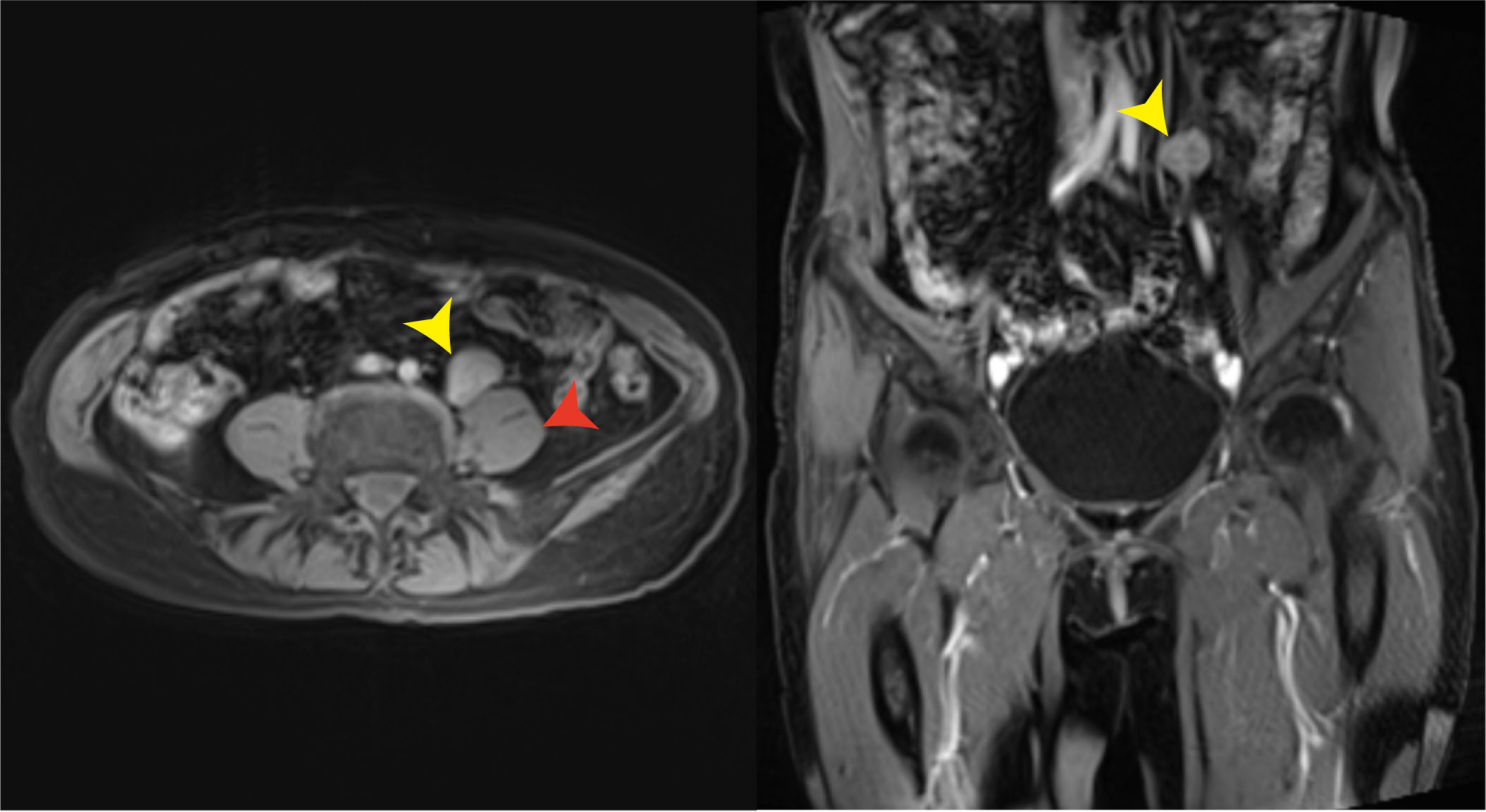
Pelvic MRI on 7 January 2022. A likely metastatic lymph node (yellow arrows) in the anterior area of the left psoas major (red arrow) and left of the common iliac artery was found 15 months after the administration of olaparib.
